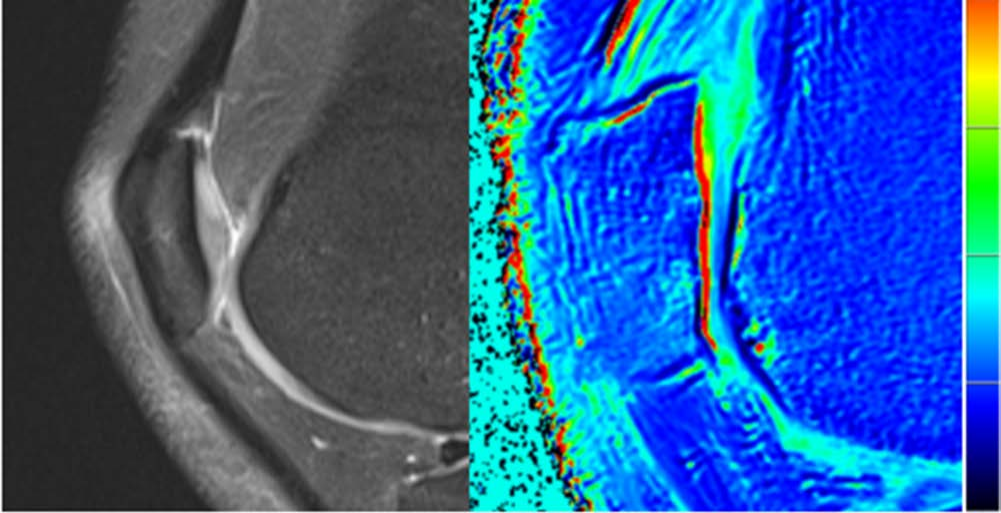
No obvious cartilage injury was found on conventional MRI and MRI functional imaging. MRI = magnetic resonance imaging.
